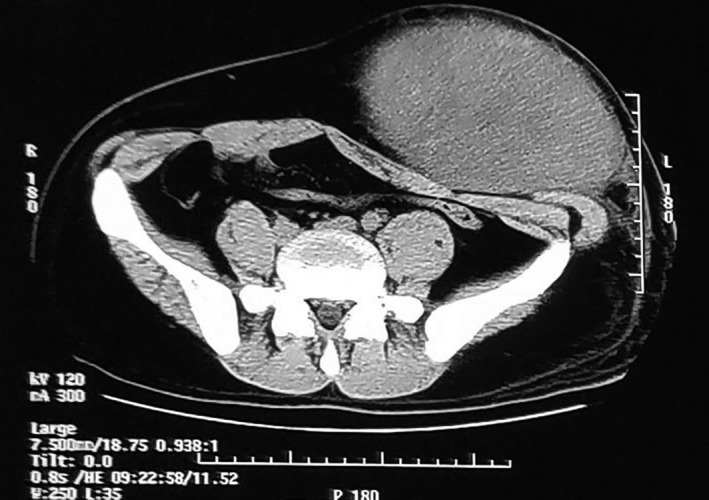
Preoperative lower abdominal computed tomography revealing soft‐tissue mass in the left abdominal wall
